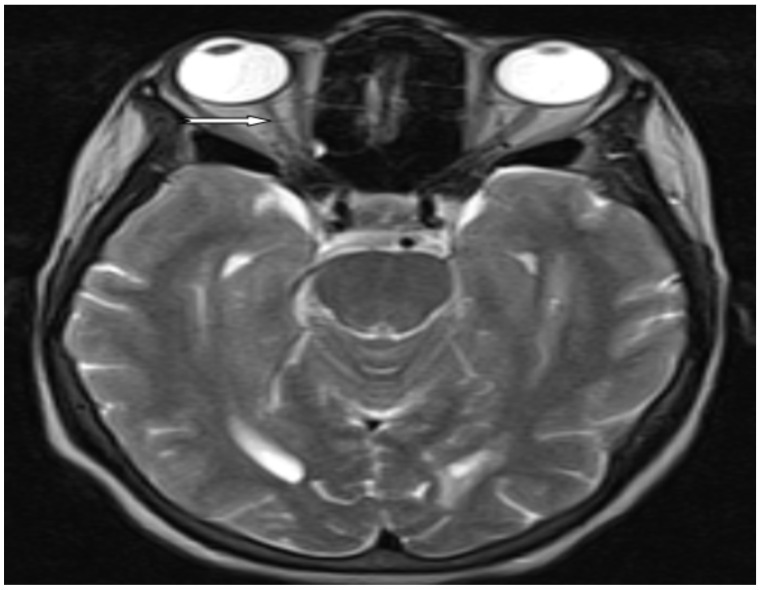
Magnetic resonance imaging studies. Axial T2-weighted Magnetic resonance imaging of the brain showed normal finding except for the presence of right side atrophied optic nerve
